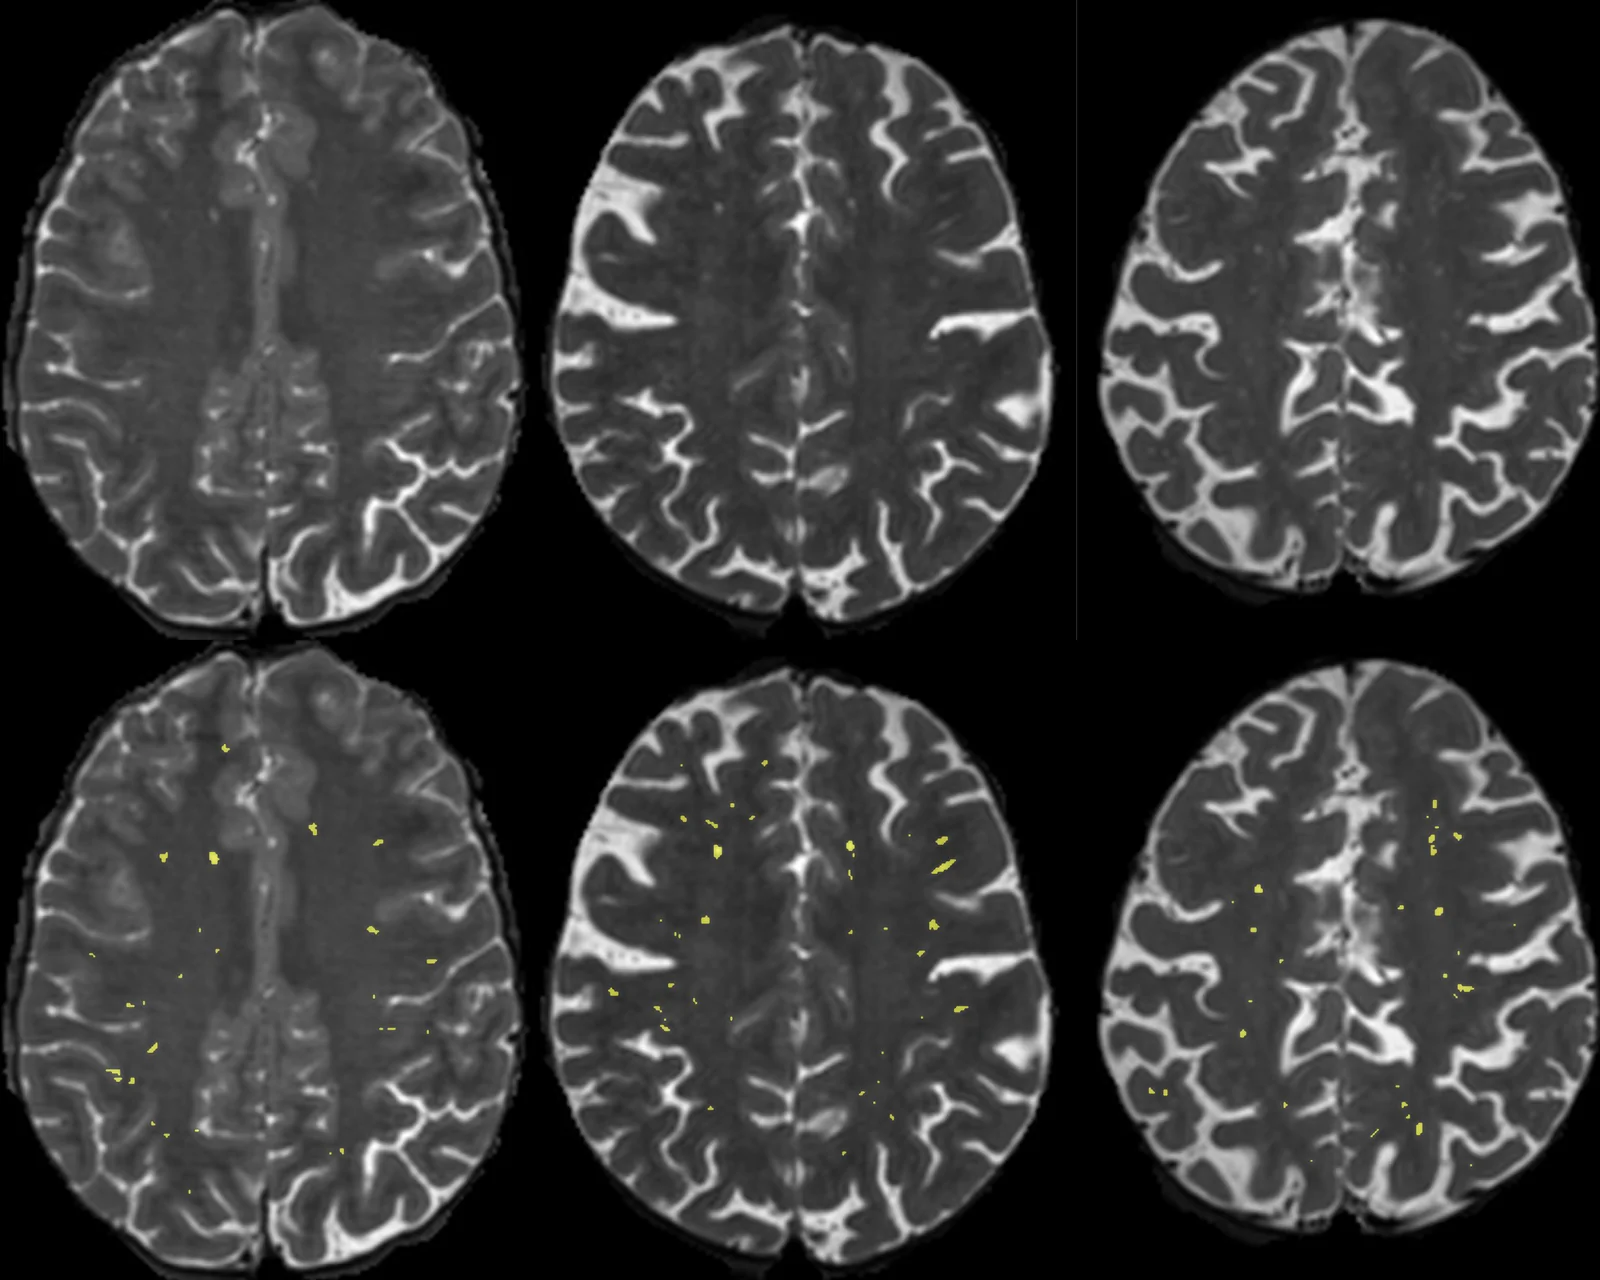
Equivalent axial T2-weighted magnetic resonance imaging slice in the centrum semiovale, with the perivascular spaces (PVS) segmented (bottom row) in yellow, in 3 consecutive generations of the same family (younger to older: left to right). Observe a similar number of PVS counted regardless of age differences, and similarities in the topological (ie, spatial) distribution patterns in consecutive generations.
